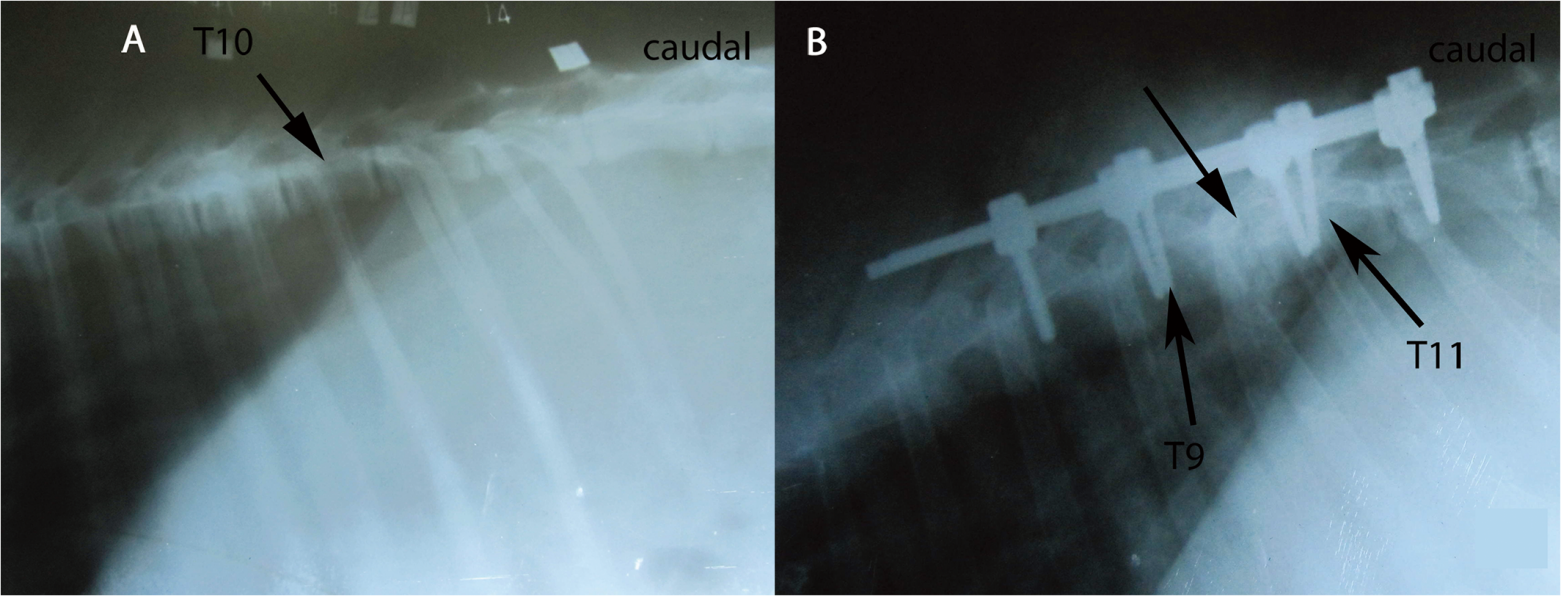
Pre- and postoperative radiographs of T8-12 with the use of a C-arm image intensifier. (A) Preoperative radiograph showing T10 marked at the 12^th^ ribs and the surface metal sign. (B) Postoperative radiograph showing that the vertebral body of T10 was resected completely and that the internal fixation was satisfactory.
